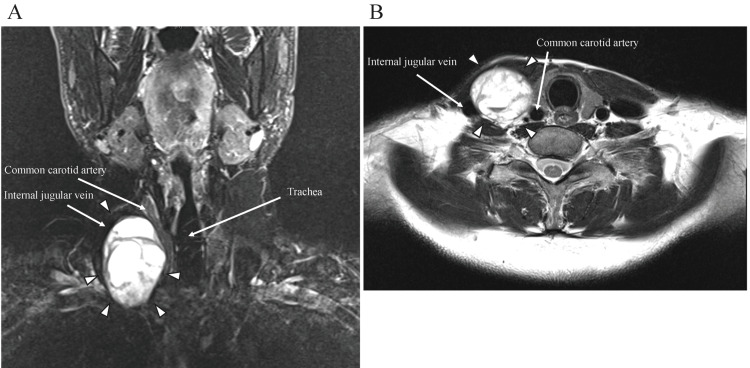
Results of magnetic resonance imaging. A: Short TI inversion recovery. B: T2-weighted. A well-defined lobulated heterogeneous mass with a split fat sign is located between the internal jugular vein and the common carotid artery (the tumor is shown surrounded by arrowheads).
